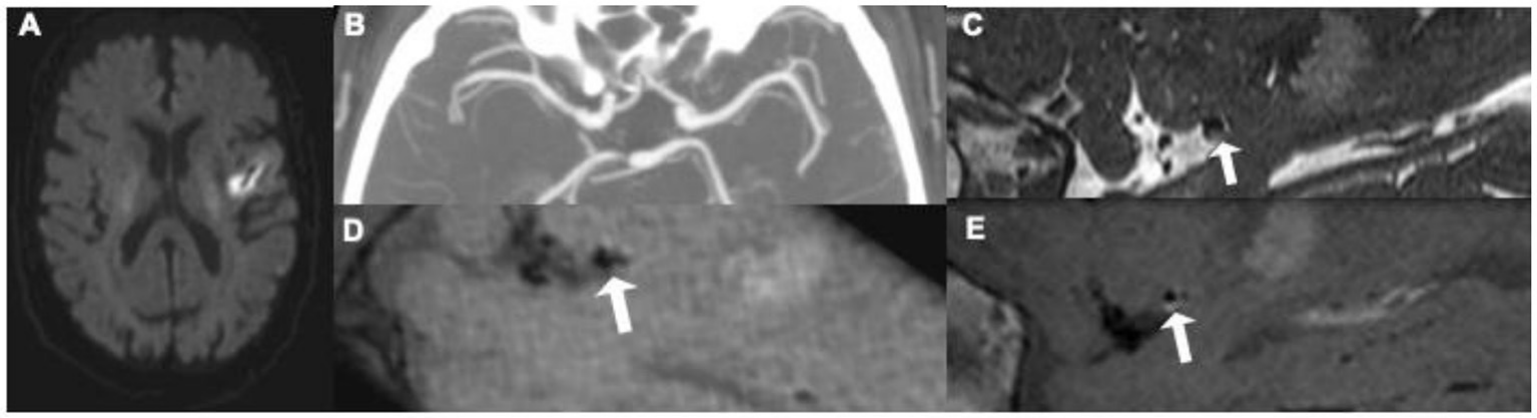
This 62-year old patient presenting with an acute left middle cerebral artery (MCA) infarction on MR (A) had CT angiography [(B) maximum intensity projection] at presentation without evidence of any significant stenosis. Initially thought to have an embolic stroke of undetermined source, he underwent an intracranial vessel wall MR where he was found to have a focal, eccentric T2 hyperintense [(C) white arrow] enhancing [(D) pre-contrast image, (E) post-contrast image, white arrow] plaque, in the distal M1 segment of the left MCA, thought to be the culprit plaque.4.
